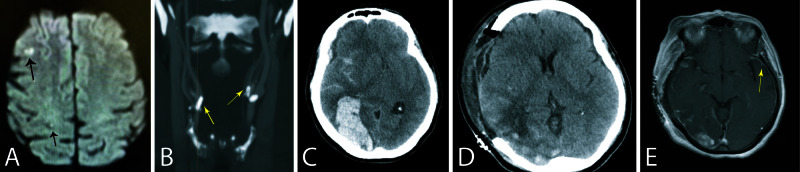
Pre- and post-operative brain images. A: Pre-operative diffusion weighted brain MRI showing previous punctate infarcts in the right middle cerebral artery territory (arrows). B: Cervical CT angiography showing bilateral dense calcified plaques at the carotid bulb and severe right internal carotid artery stenosis (arrows). C: Post-endarterectomy brain CT scan showing a large temporo-occipital intraparenchymal hemorrhage, subarachnoid, and subdural hemorrhage. D: Brain CT scan after decompressive craniectomy and clot evacuation. E: Post-endarterectomy gadolinium enhanced brain MRI showing clot evacuation and diffuse leptomeningeal enhancement on postdecompression day 6 (arrow).
